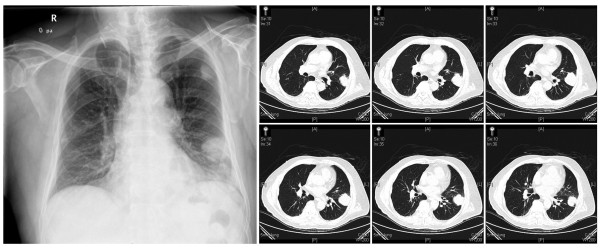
Preoperative CT scan of the left elbow revealing signs of metastatic tumor along with destruction and pathologic fracture of the olecranon.
